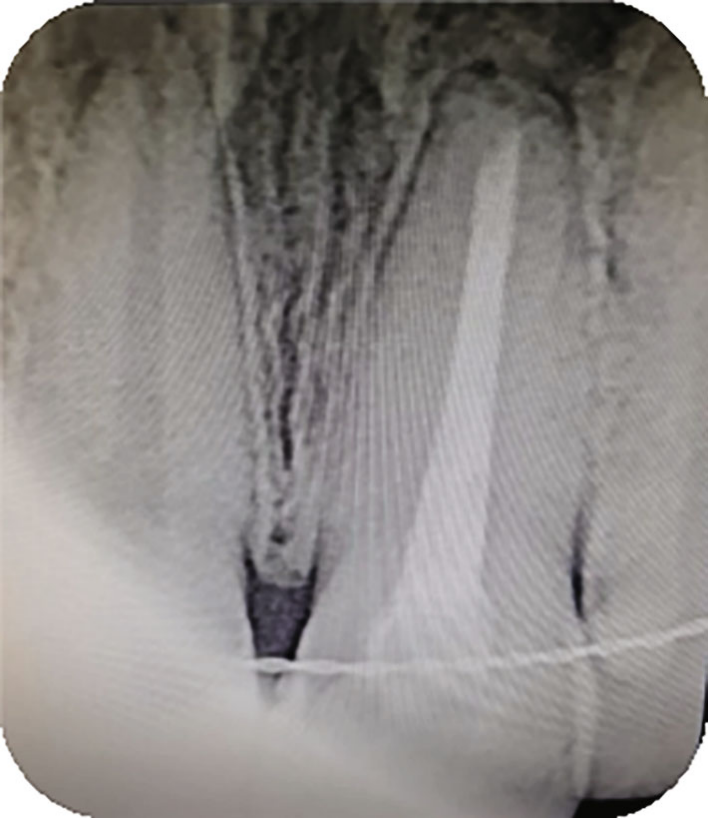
Radiographic examination 12 months after apical surgery.
